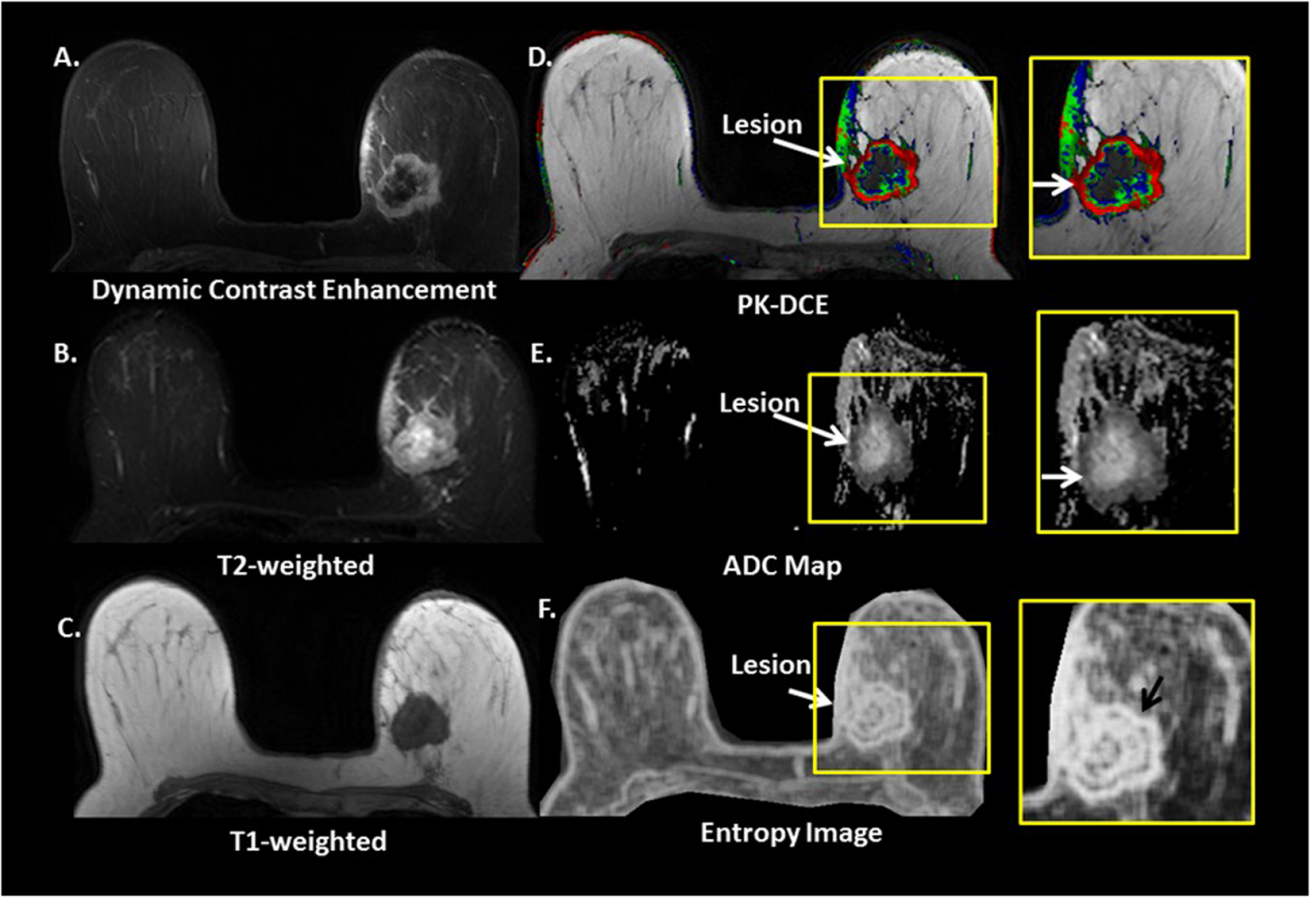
Typical multiparametric breast image of a malignant patient. a Dynamic contract enhanced, b T2-weighted, c T1-weighted, d Pharmacokinetic-DCE (PK-DCE) overlay of *K*
